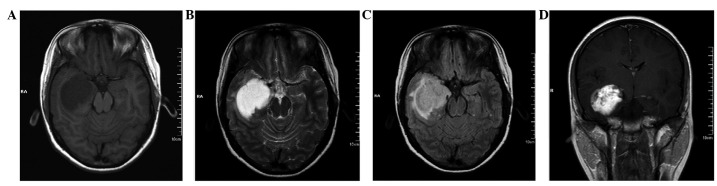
Pre-operative MRI of the initial surgery. (A) A hypointense well-defined lesion in the right temporal lobe, shown by T1WI. (B) A homogeneous hyperintense lesio, shown by T2WI. (C) A heterogeneous hyperintense lesion with slight edema, shown by FLAIR sequence imaging. (D) Patchy enhancement of the tumor, shown by contrast-enhanced imaging. MRI, magnetic resonance imaging; WI, weighted imaging; FLAIR, fluid-attenuated inversion recovery.
